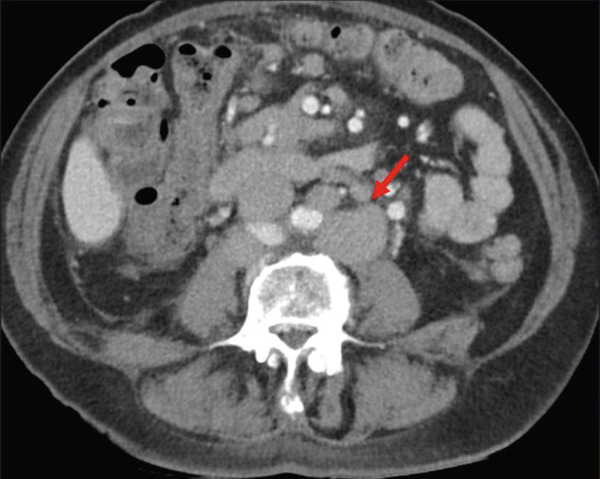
Lymphadenopathy in the para‐aortic lymph nodes seen on contrast‐enhanced CT; biopsy confirmed follicular lymphoma.
